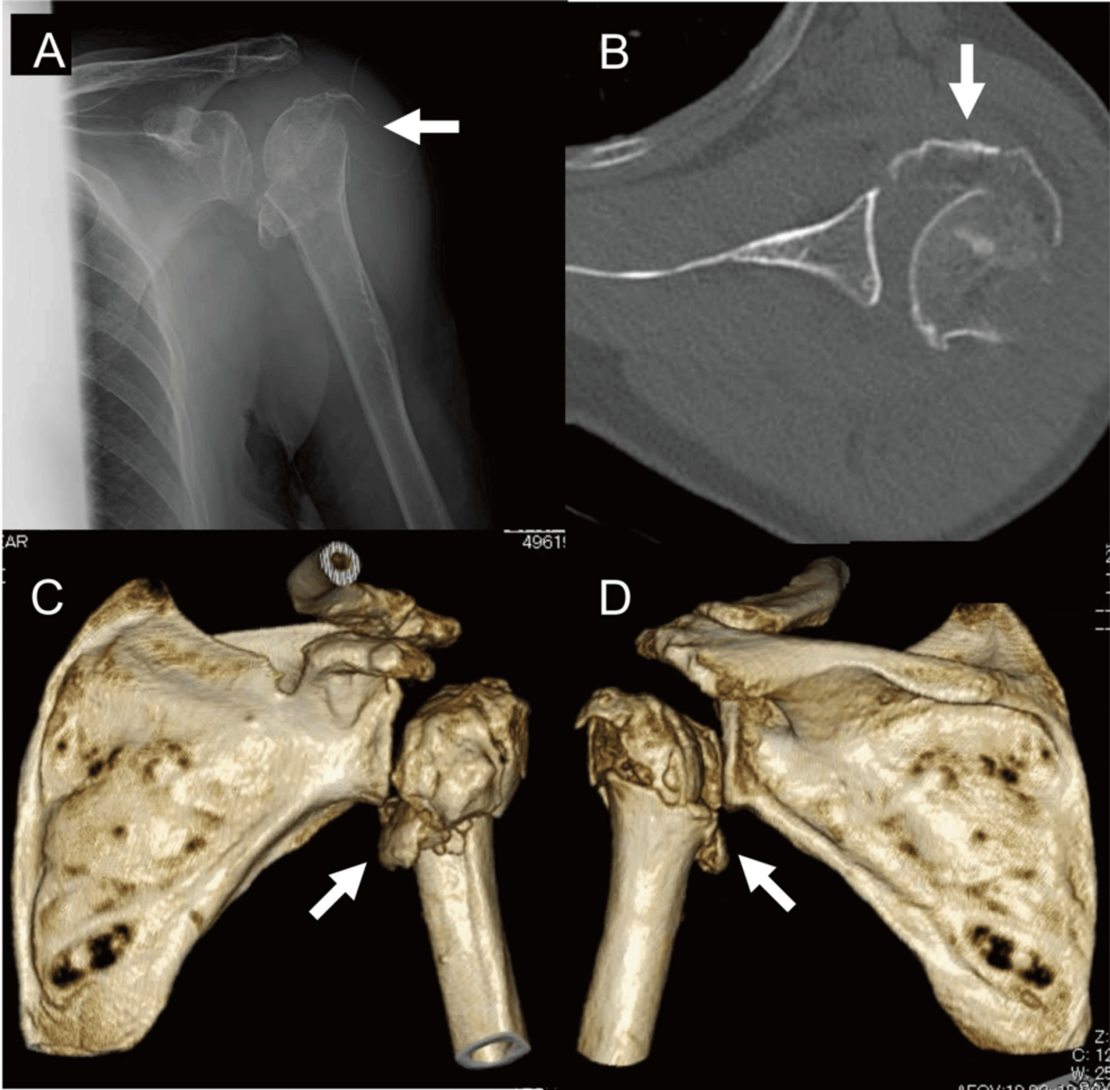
The preoperative images of the left shoulder. The preoperative images of the left shoulder include an anteroposterior X-ray (A), an axial view of CT (B), an anterior view of 3D-CT (C), and a posterior view of 3D-CT (D). These images show a proximal humeral fracture (indicated by an arrow) with osteoarthritis (OA).
